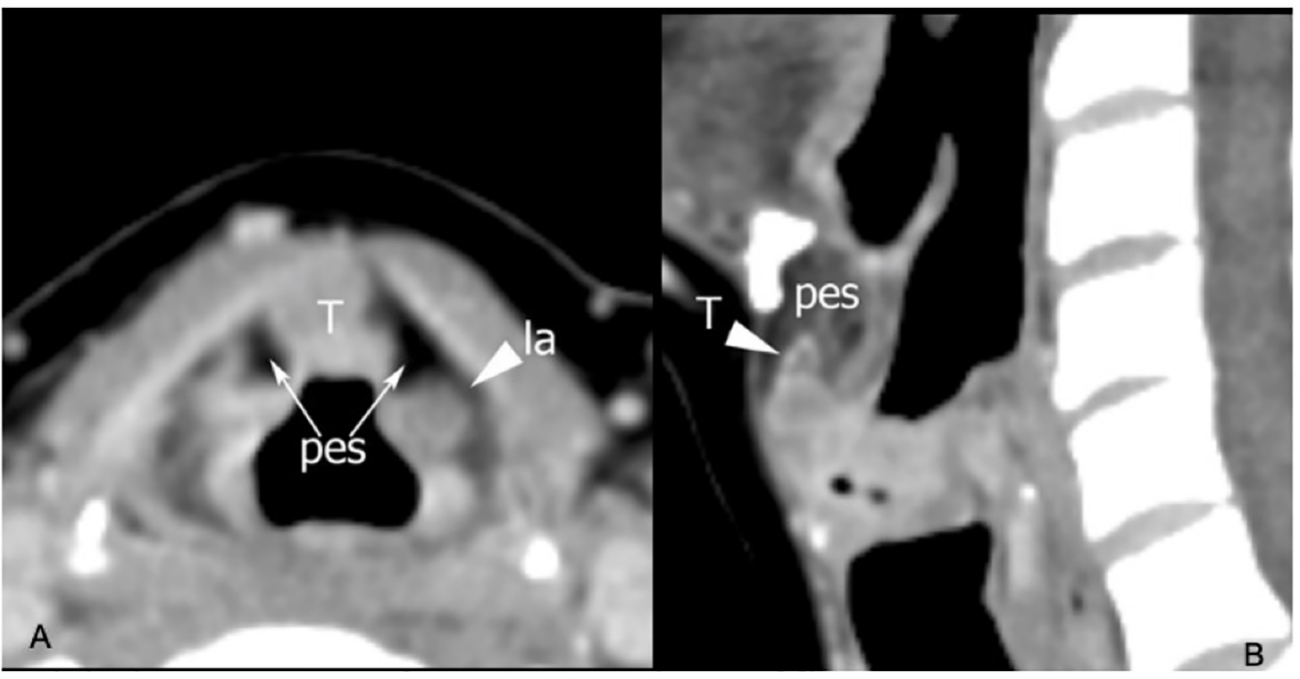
CT on axial plane (A) at the supraglottic level identifies tumoral tissue (T) involving the median part of the pre-epiglottic space (PES), while the lateral portions of this visceral space (arrows) show regular fat density. Arrowhead points a laryngocele (la) in the left superior paraglottic space. On sagittal plane (B) the lesion invades the inferior part of the PES.
